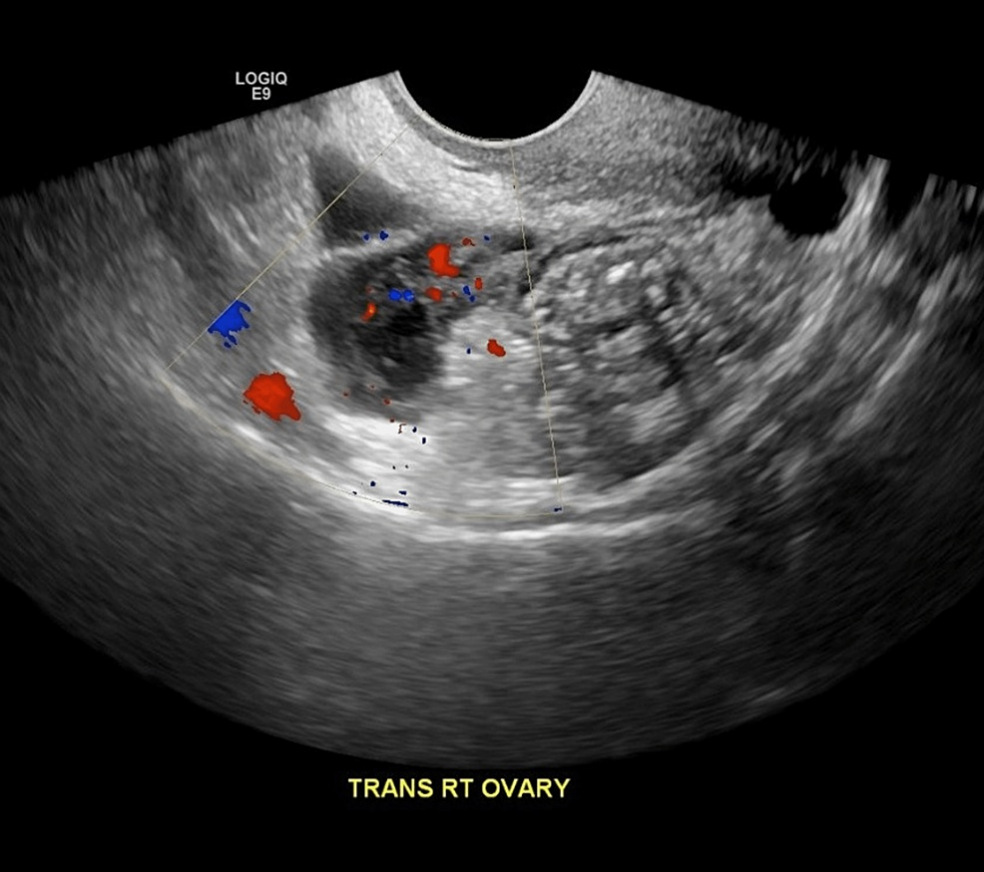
Transabdominal ultrasound demonstrates a right TOA measuring 6.2 x 3 x 2.5 cm. TOA: tubo-ovarian abscess
